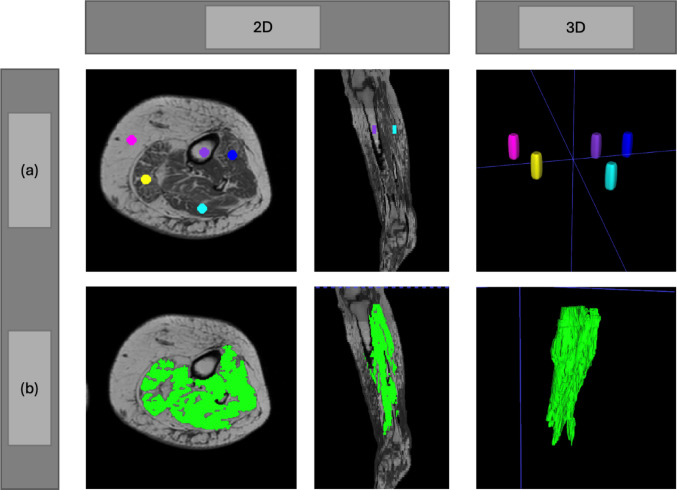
Semi-automated muscle segmentation and region-of-interest (ROI) placement in lower leg MRI. Example of image analysis in a patient with hereditary neuropathy (CMT1C). (a) ROI placement (colored circles) in muscle, fat, and bone marrow on an axial T1-weighted image (left), with the corresponding sagittal reconstruction (center) and 3D representation of the ROI positions (right). (b) Semi-automated segmentation of lower leg musculature (green overlay) performed with ITK-SNAP on axial slices (left), sagittal reconstructions (center), and 3D renderings (right). These approaches were used to quantify muscle volume, normalized T1 signal intensity (nSI), and proton density fat fraction (PDFF)
